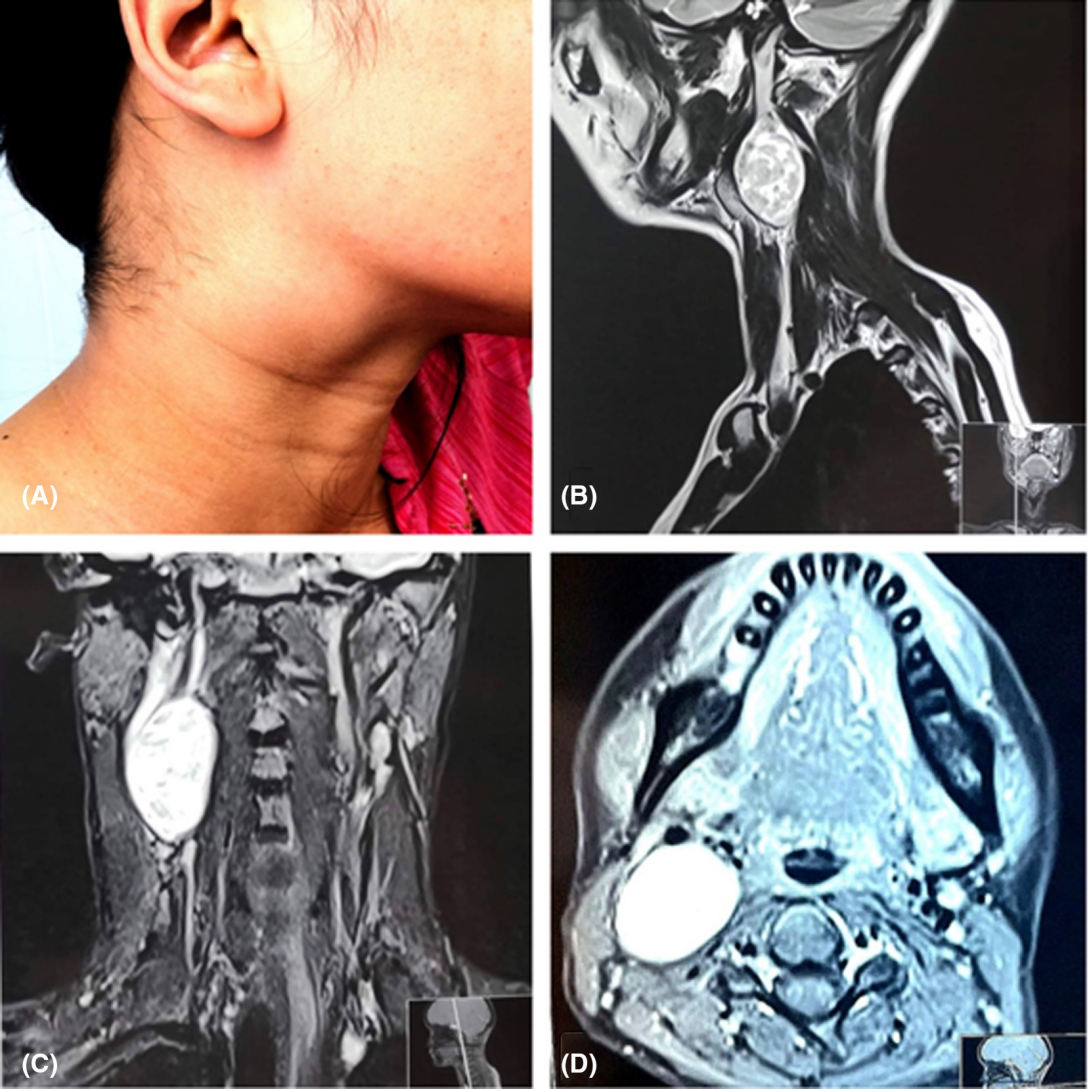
(A) Swelling noted in neck, (B–D) MRI T2 Sagittal, coronal, and axial section respectively showing well defined lobulated lesion (2.4 × 2.4 × 2.8 cm), heterogeneous signal changes in right lateral neck in carotid space. The lesion is displacing the internal carotid artery and internal jugular vein anteriorly without luminal narrowing. Laterally the lesion is abutting sternocleidomastoid muscle with maintained fat plane.
